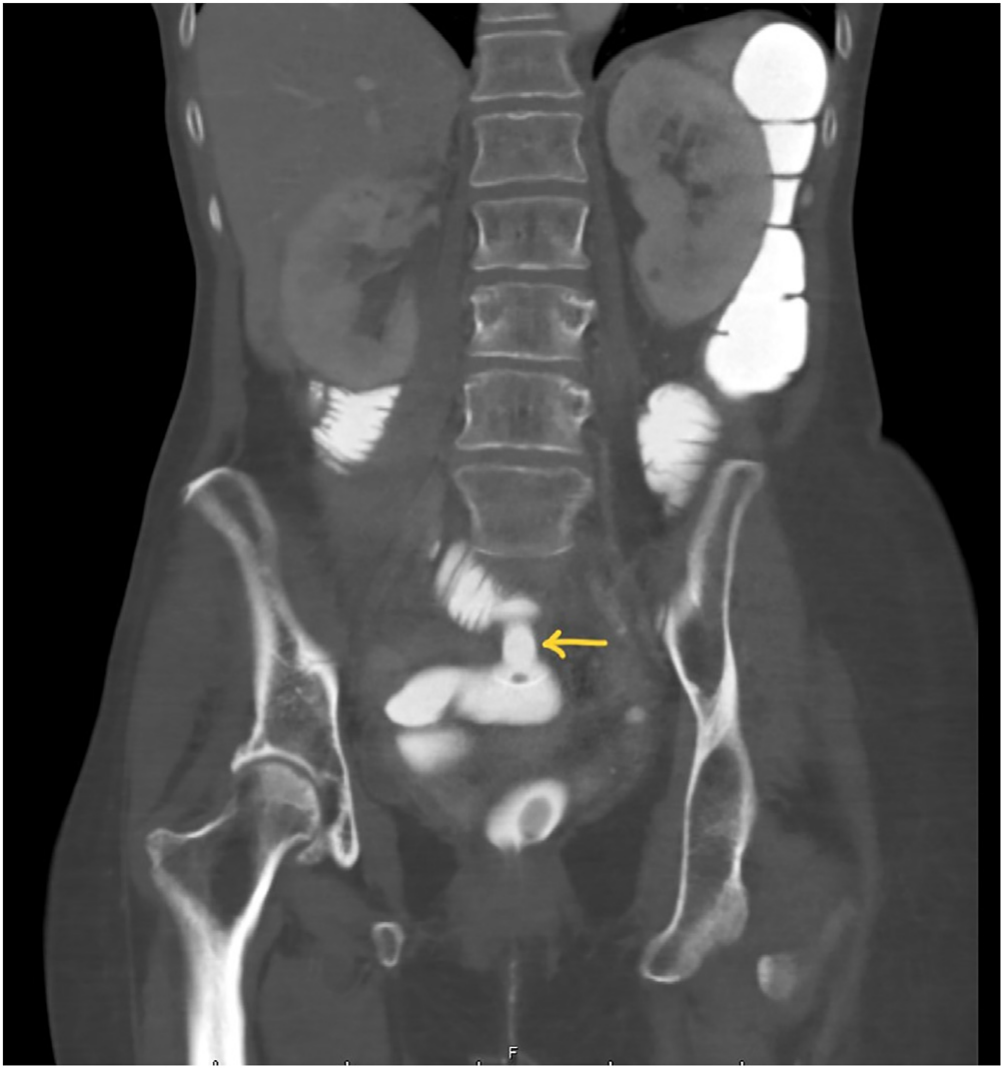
Coronal CT image of the ileocolostomy (*yellow arrow*) and decompression of the small bowel.
